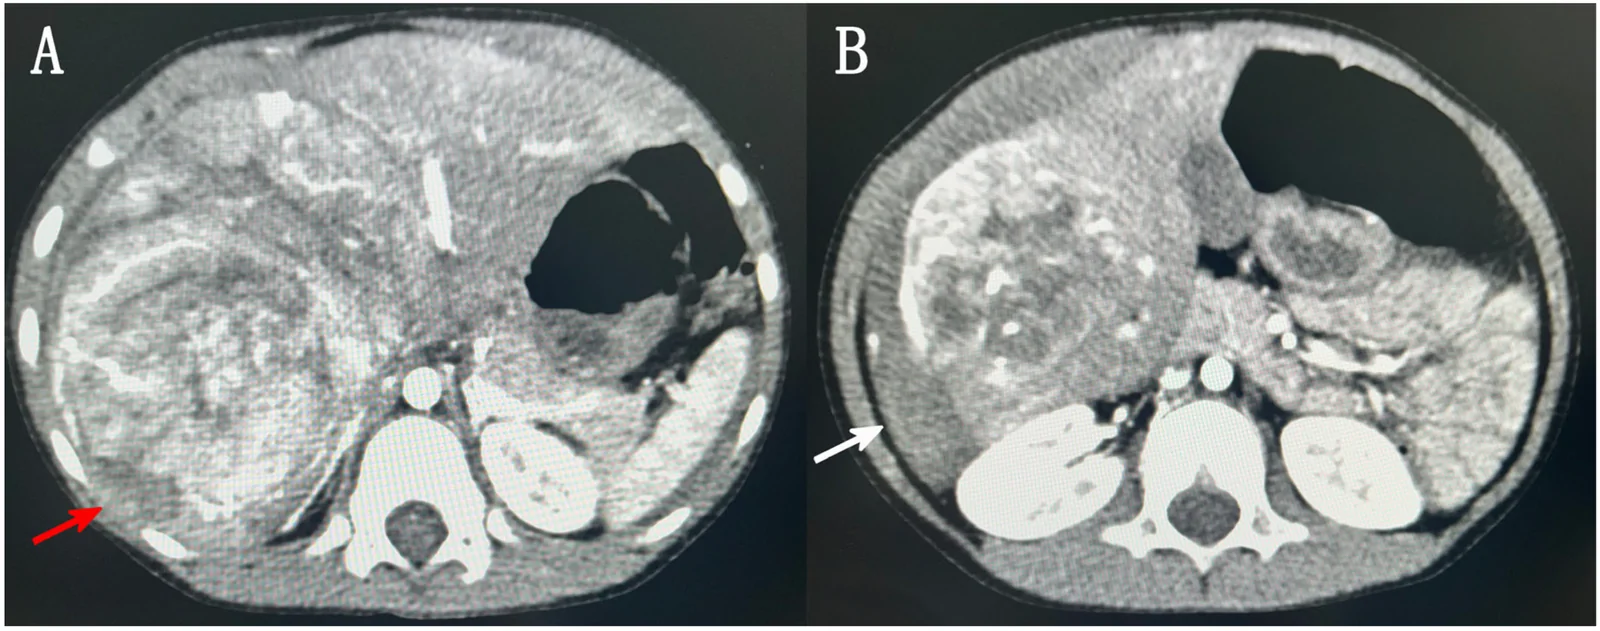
Contrast-enhanced computed tomography findings of spontaneous hepatoblastoma rupture: (A) Large hepatic mass with focal capsular discontinuity (red arrow). (B) Peritumoral and intraperitoneal fluid compatible with hemorrhage (white arrow).
